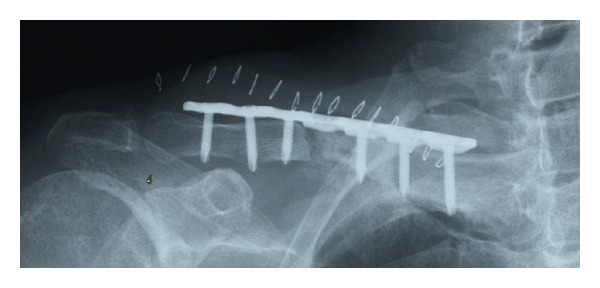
Postoperative radiograph demonstrating fracture fixation with 7-hole locking plate supplemented with autologous bone grafting.
